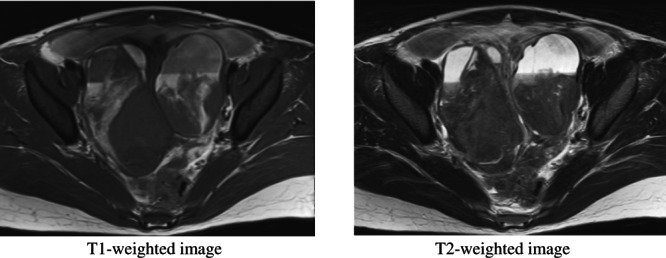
Pelvic MRI findings. In the early stage of a hemorrhage, ovarian endometriotic cysts contain different stages of clotting and therefore present with a variety of MRI signals.
